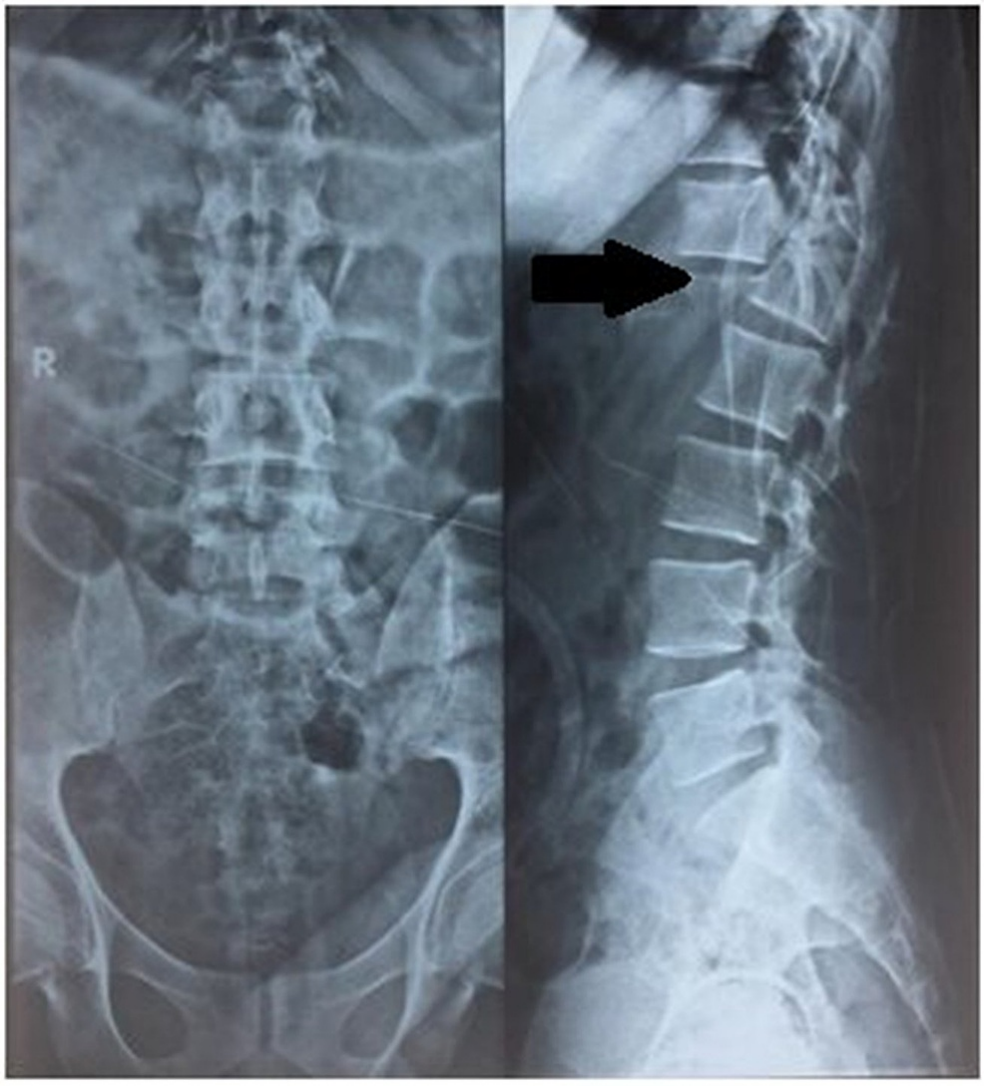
Preoperative radiograph (AP and lateral) of thoracolumbar spine showing T12-L1 fracture-dislocation (black arrow) AP - anteroposterior
